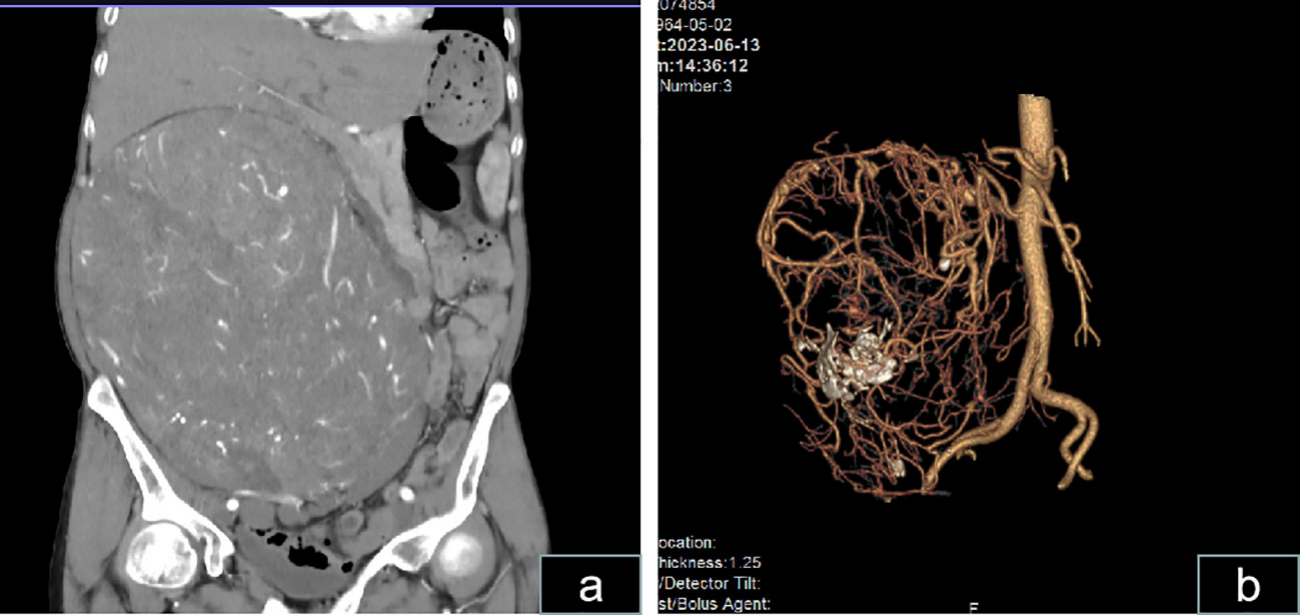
Computed tomography scans show a massive abdominal mass. (A) CT scan revealing a massive abdominal mass with dimensions of approximately 25.8 × 22.1 × 15.6 cm exhibiting irregularity and enhancement is shown. (B) Vascular reconstruction revealing an extremely rich blood supply for the mass and the presence of a few calcifications within the mass is shown.
